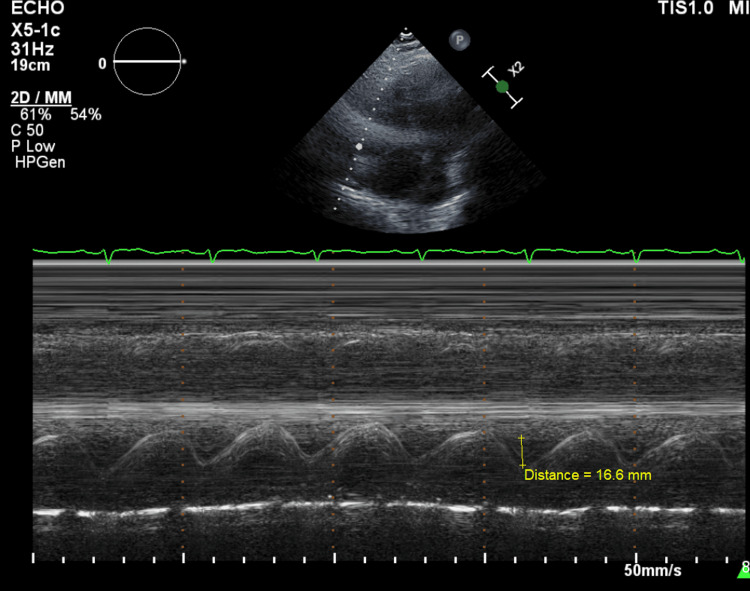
M-Mode Echocardiography from an Apical Four-Chamber View Measurement of tricuspid annular plane systolic excursion (TAPSE) demonstrating a value of 16.6mm indicating right ventricular dysfunction.
